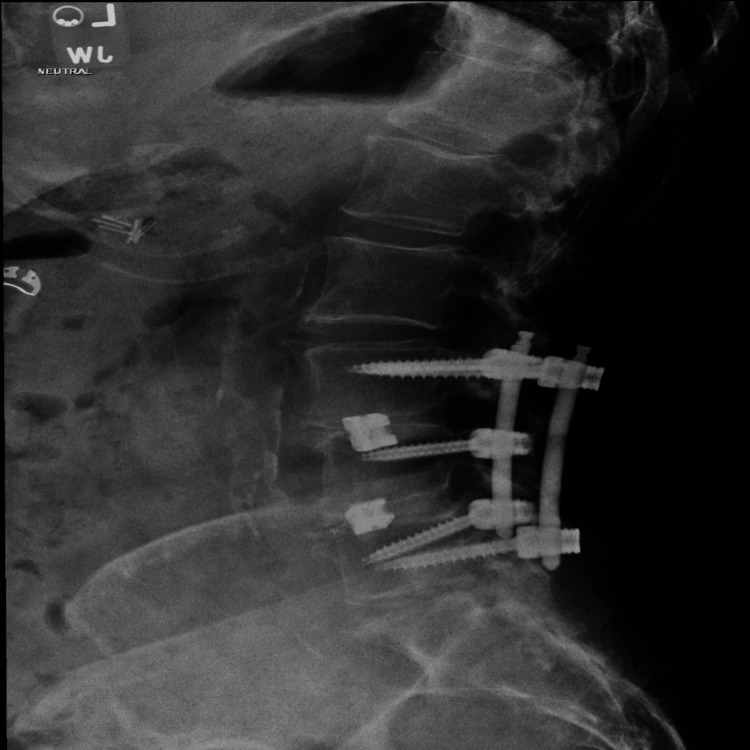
An X-ray of the lumbar spine (lateral view); left transforaminal lumbar fusion L3-L5 is visualized.
